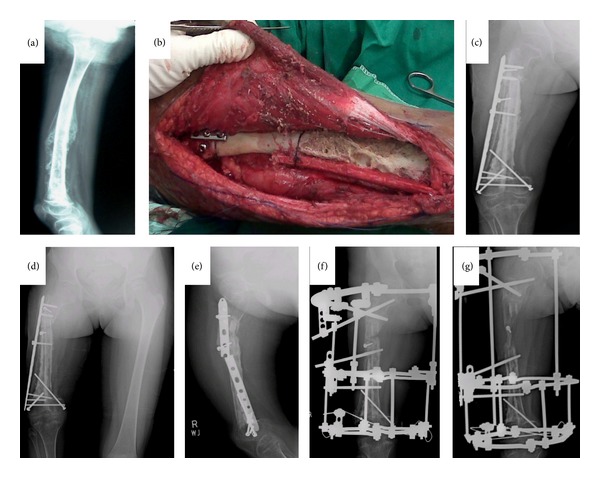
A 10-year-old girl with Ewing's sarcoma. (a) X-ray shows mass in mid and distal right femur. (b) Intraoperative picture showing autoclaved bone with fibular graft. (c) Immediate postoperative X-ray (d) 26-month postoperative X-ray shows graft and bone union at both distal and proximal ends; however, there is considerable shortening of right femur. (e) Breakage of locking compression plate (f) Considering the limb length discrepancy and plate breakage, patient underwent Ilizarov application to right femur. (g) One year post-Ilizarov X-ray showing 2.5 cm gain in right femur length.
